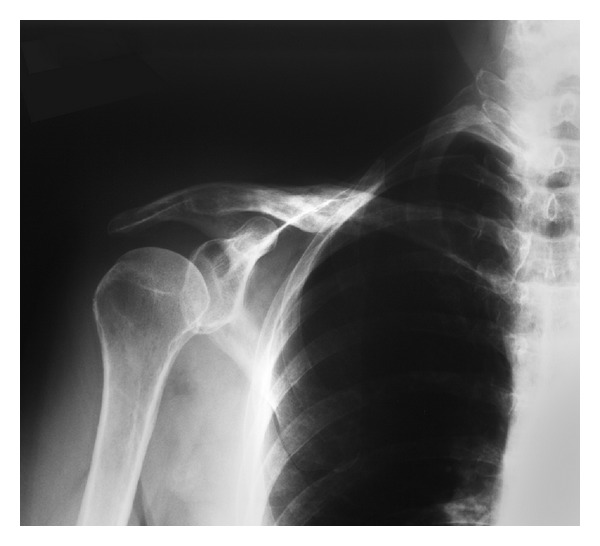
Radiograph of the healed right clavicle fracture after hardware removal.
